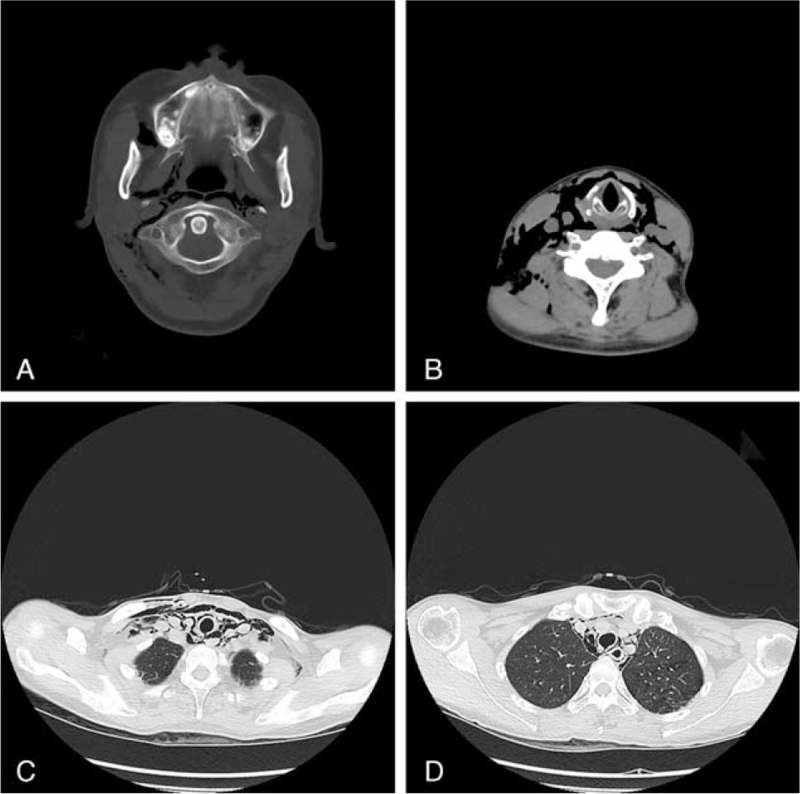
(A and B) The CT shows subcutaneous emphysema from the base of the skull to the chest; (C and D) tear on the tracheal wall from 4.5 to 8.5 cm under the glottis.
